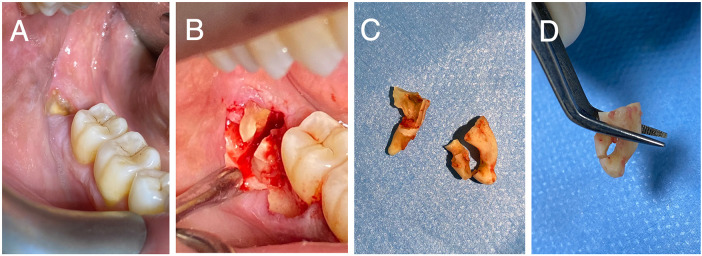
(A) Intraorally exposed root observed two years after the initial coronectomy. (B) Segmentation of the remaining M3 into mesial and distal root segments. (C) All extracted root fragments. (D) Close-up view of the retrieved mesial root fragment after sectioning, demonstrating the apical channel-like space between the fused mesial root components corresponding to the pathway of IAN observed in CBCT.
